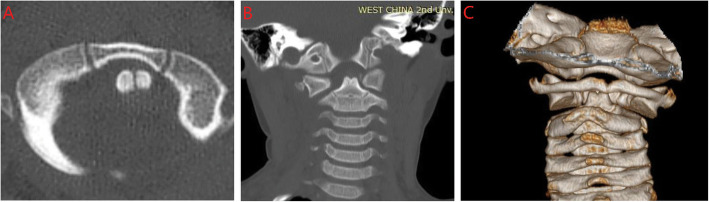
An axial computed tomography (CT) scan shows atlantoaxial rotatory subluxation (a and b). A three-dimensional CT scan showing subluxation of the C1-C2 cervical tract (c)
